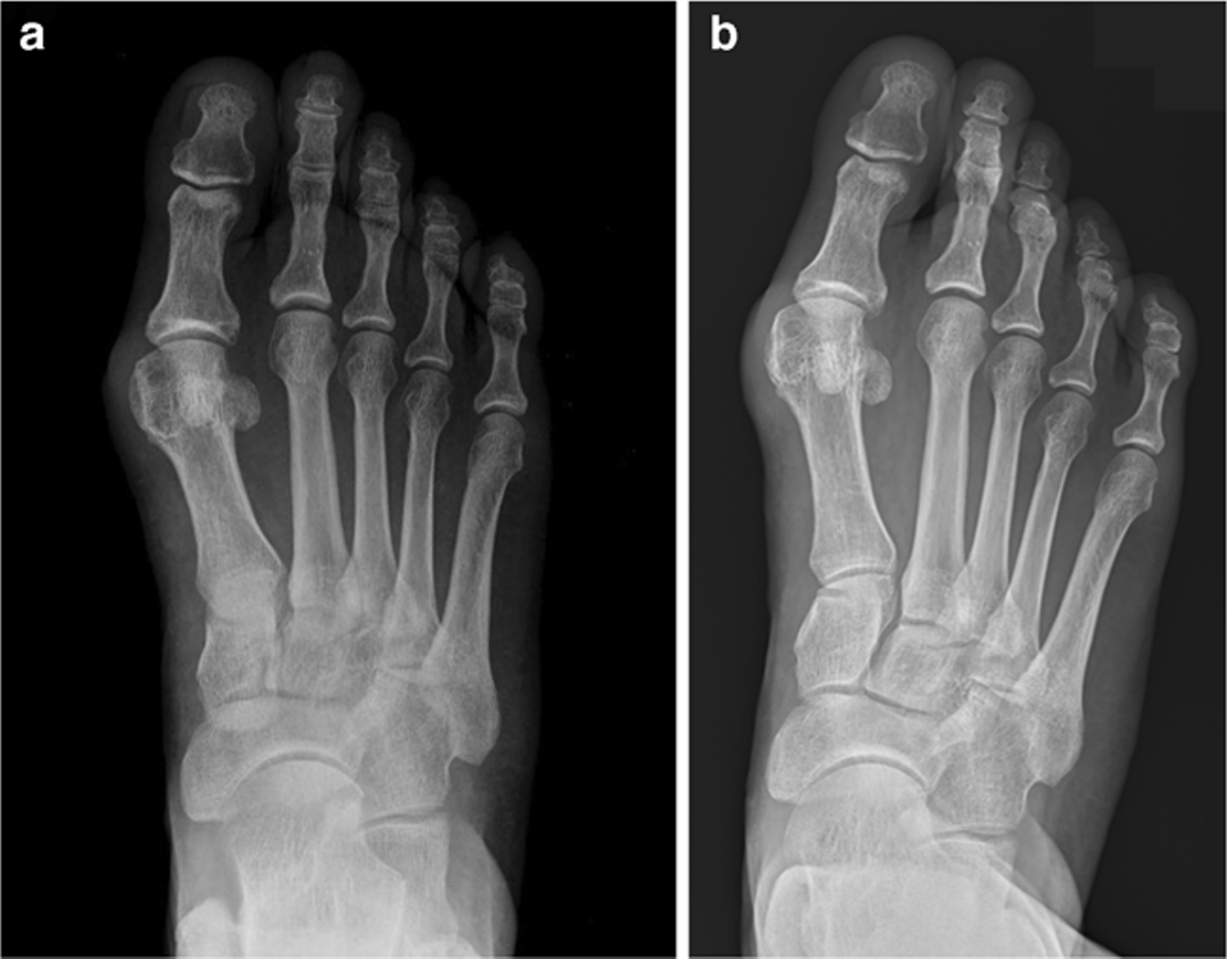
The non-weightbearing (a) and weightbearing (b) x-rays of the same patient. A 5° increase in HVA and 2.6° increase in IMA were observed
